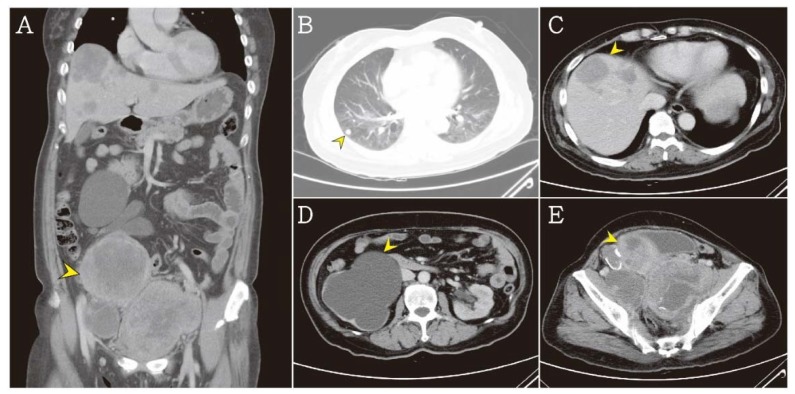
(A) The abdominal computed tomography revealed a huge heterogeneous density mass (arrow head). (B) Multiple pulmonary nodules were noted in lung field (arrow head). (C) Multiple heterogeneous hepatic tumors were noted (arrow head). (D) Severe hydronephrosis at right kidney were noted due to tumor compression (arrow head). (E) Three parts of heterogeneous pelvic tumors were noted about 9.4 × 8.6 × 9.3 cm^3^, 7.1 × 6.1 × 6.0 cm^3^, 11.1 × 7.5 × 9.1 cm^3^ (arrow head).
